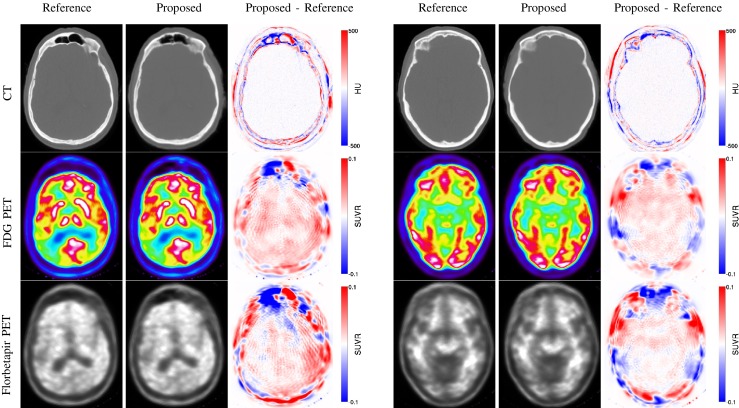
Example of CT (top row), ^18^F-FDG PET (middle row) and ^18^F-florbetapir PET (bottom row) images obtained from the reference and pseudo CTs, and difference images, for an amyloid positive (left) and an amyloid negative (right) subjects
